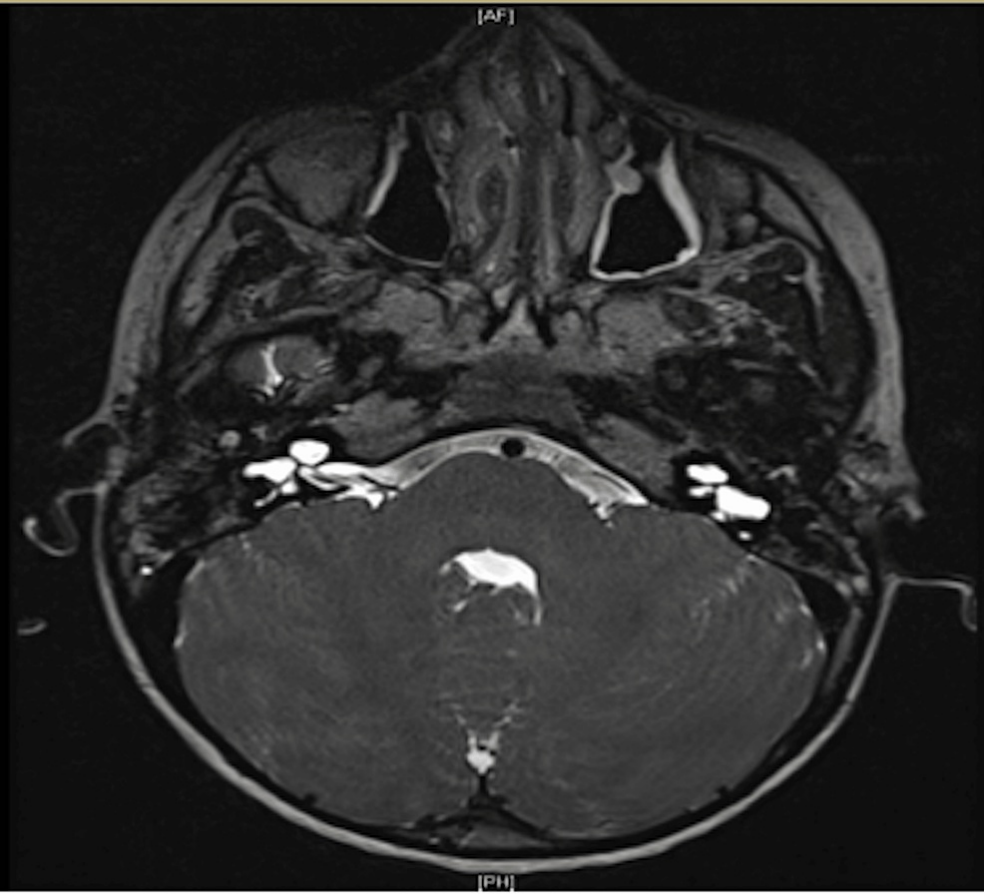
MRI T2-weighted image at the level of internal auditory canal showing atresia of the left internal auditory canal with no identifiable cochlear nerve. Right cochlear nerve is identified passing through the right auditory canal. Bilateral cystic vestibule and bilateral cystic apical and middle turns of the cochlea.
